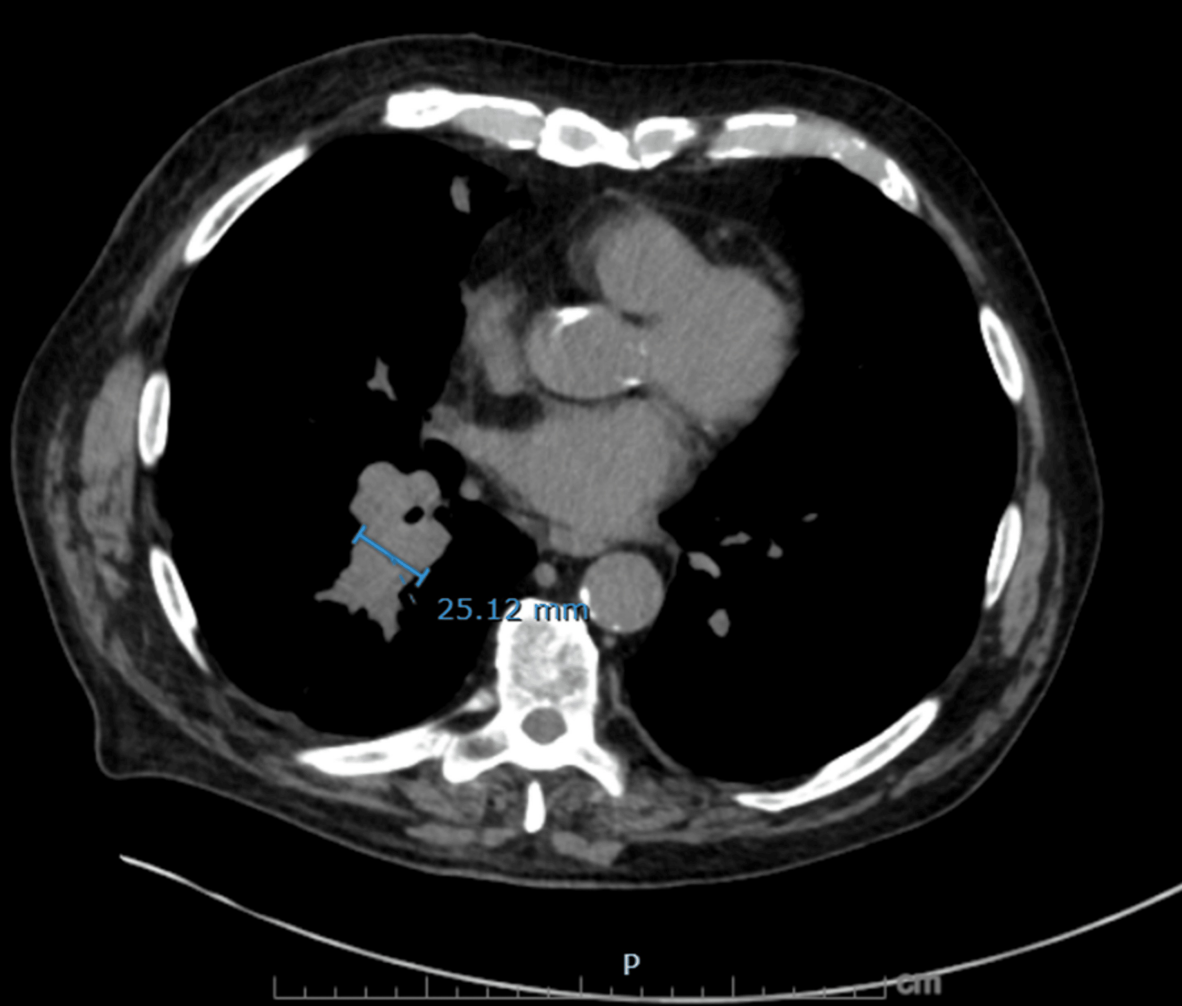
Axial non-contrast CT chest showing right infrahilar mass-like fullness Soft tissue density in the right lower lobe infrahilar region measuring up to 25 mm in transverse diameter, concerning for primary malignant involvement.
